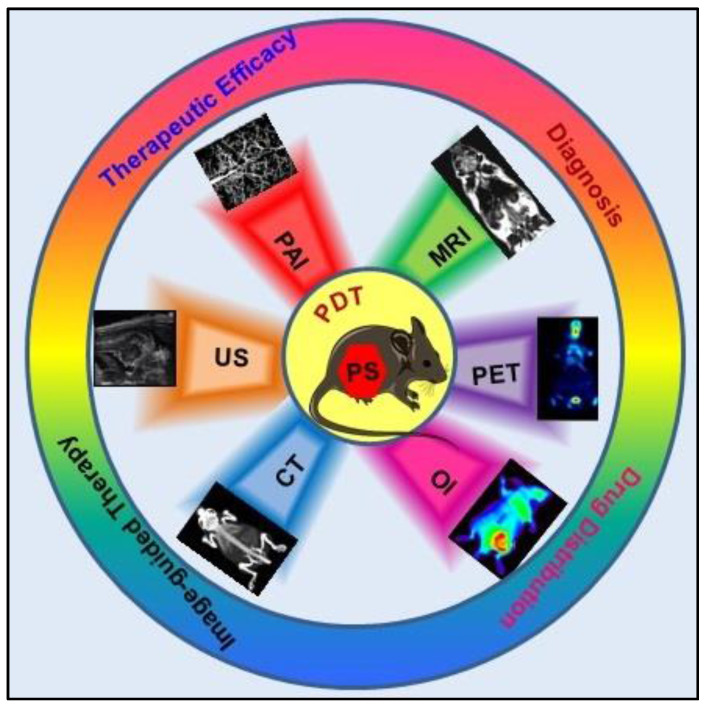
Theranostic Photosensitizers: Applications of theranostic photosensitizers (PS) in various imaging modalities: Magnetic Resonance Imaging (MRI), Positron Emission Tomography (PET), Optical Imaging (OI), X-ray computerized tomography (CT), Ultrasound Imaging (US), Photoacoustic Imaging (PAI).
